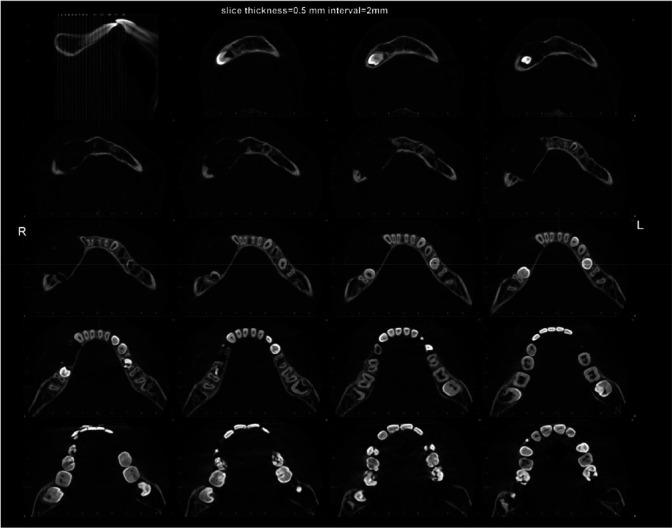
Axial view of CBCT shows thinning and expansion of both buccal and lingual cortical plates at the lesion site
